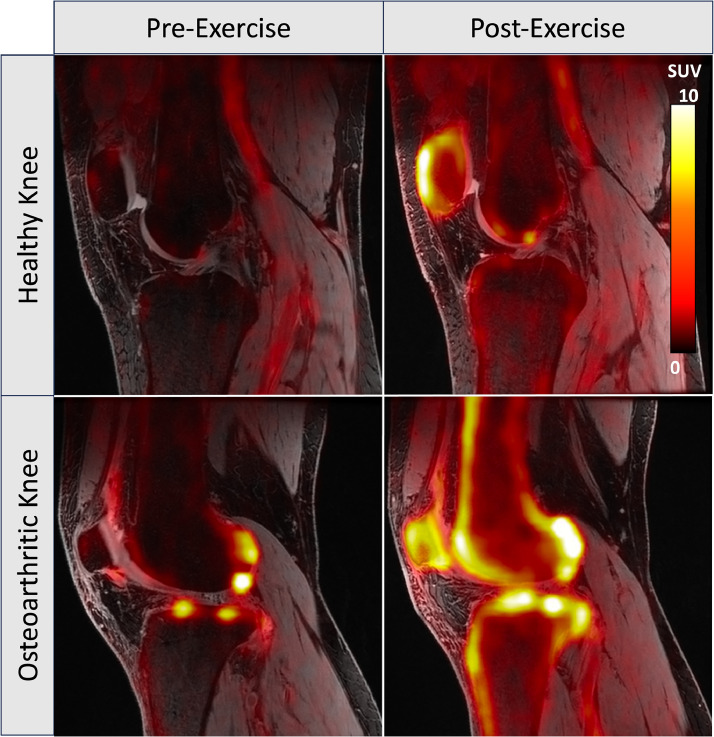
Representative fused [^18^F]NaF PET-MR images of a healthy knee (top row, 29-year-old male) and a knee with osteoarthritis (bottom row, 42-year-old male). The baseline (“Pre-Exercise”) images are shown on the left, while images acquired after a stair-climbing activity (“Post-Exercise”) are shown on the right. Both knees show increased uptake after the stair-climbing exercise, particularly in the anterior patella and the tibial tuberosity, which undergo large stresses from the patellar tendon. In the OA knee, however, multiple focal areas of increased uptake are observed, both at baseline and after exercise, representative of underlying structural pathology associated with OA.
